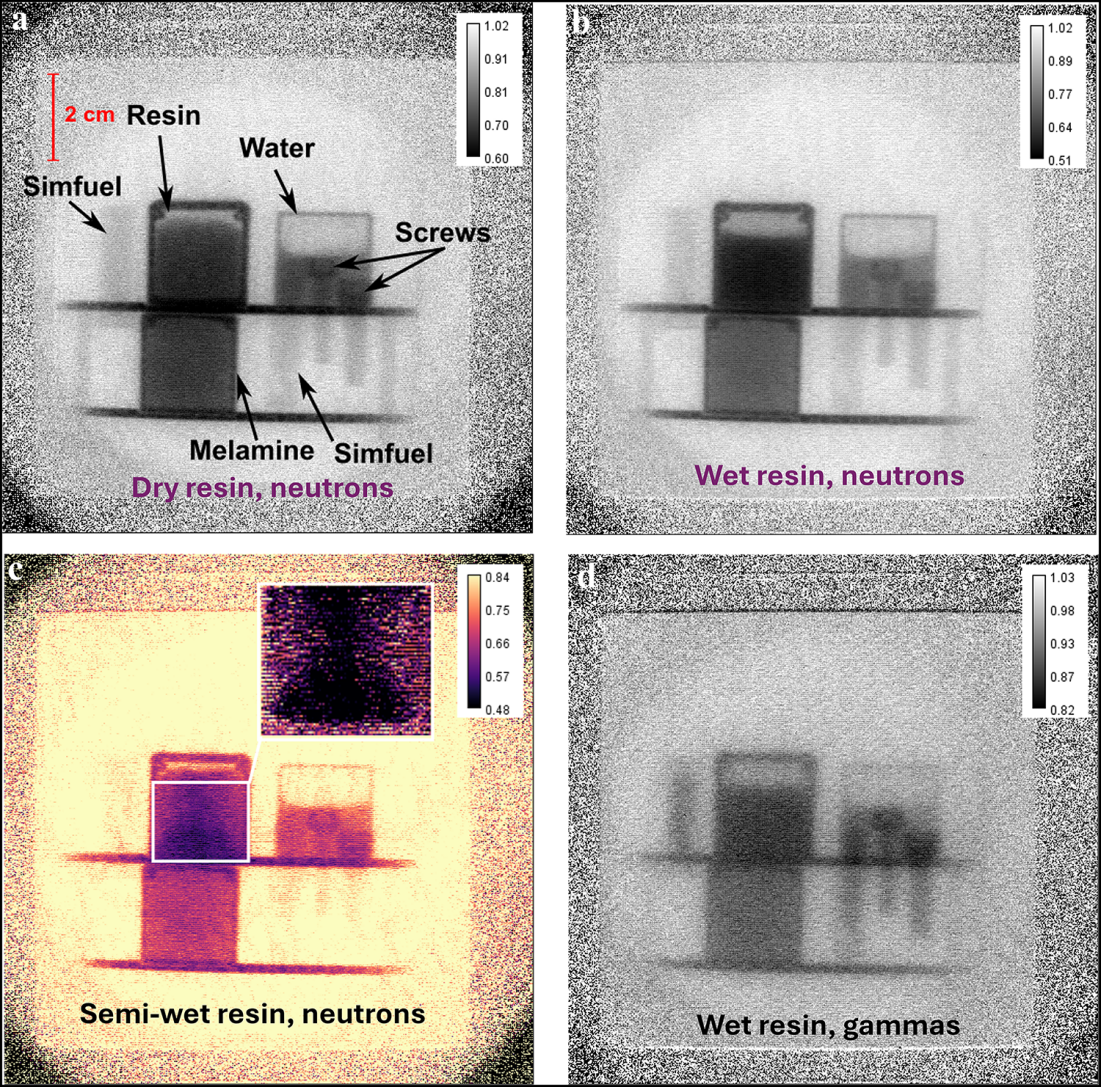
Radiographs of the sample described in “Experimental setup” (a) with dry, (b) wet, and (c) semi-wet ion exchange resin, made with 2.5–8 MeV neutrons. A radiograph of the wet resin setup made with gammas is shown in d). The enlarged window in (c) shows the difference image of the semi-wet and the dry resin (normalized semi-wet - normalized dry) for the region of interest marked white.
